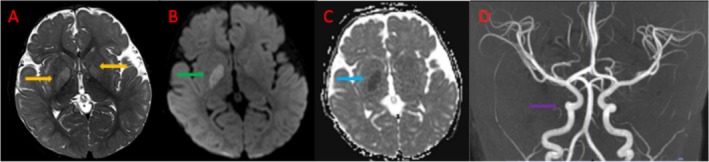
MRI and MRA Findings at age 9 months during the acute episode. (A) Axial T2‐weighted MRI showing T2‐signal hyperintensity of the globus pallidus bilaterally (right more than left) (orange arrows). (B) Axial diffusion weighted imaging (DWI) showing diffusion restriction of the right globus pallidus (green arrow). (C) Axial apparent diffusion coefficient (ADC) MRI showing a decrease in ADC value correlating with DWI changes (blue arrow). (D) MRA reconstruction showing relative tortuosity of the internal carotid arteries at the skull base (purple arrow).
